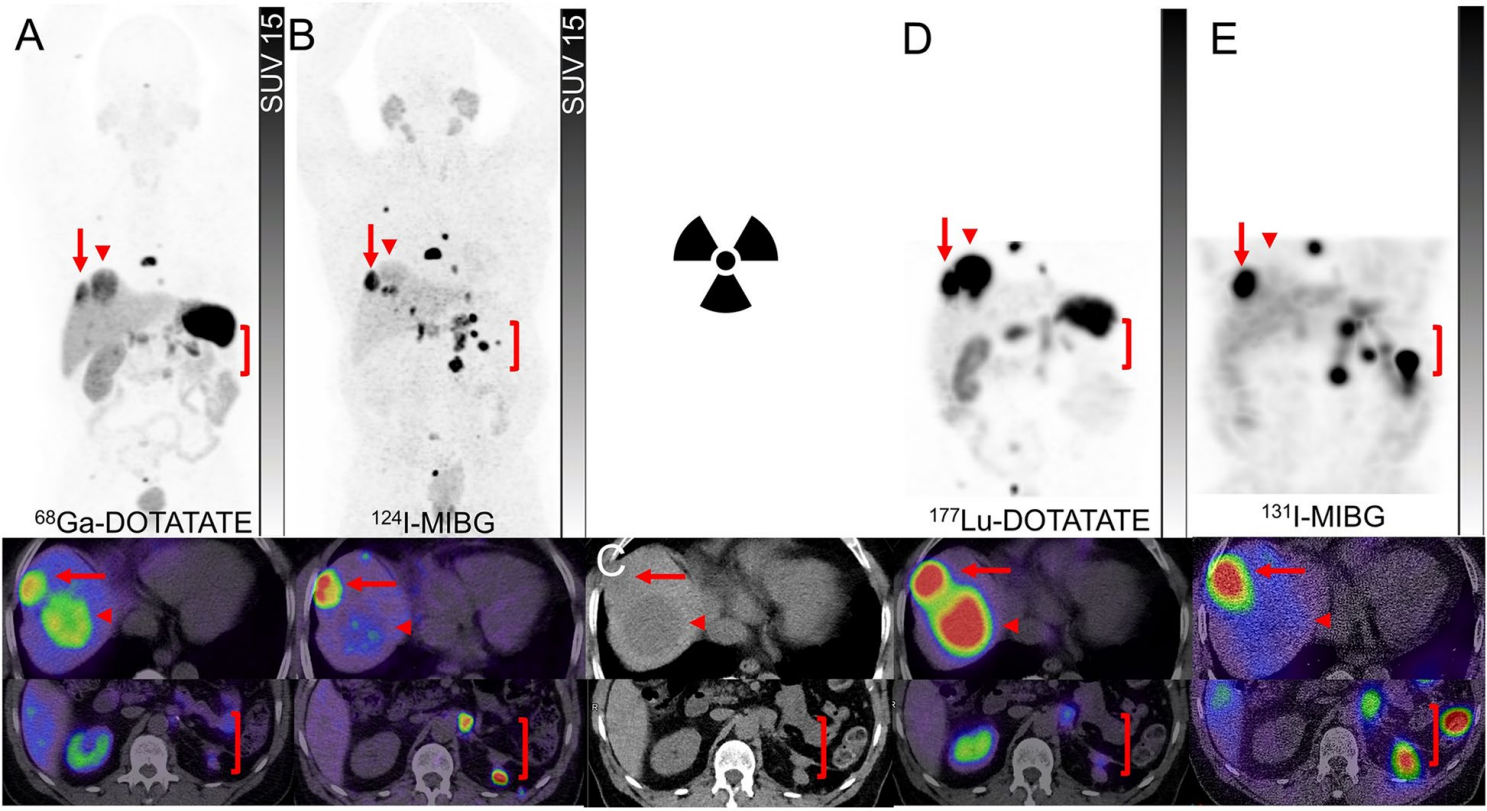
A patient with metastatic pheochromocytoma, previously treated with left adrenalectomy, presented with rapidly rising metanephrines. ^68^Ga-DOTATATE PET and PET/CT(A) showed intense uptake (Krenning score 4) in a thoracic vertebra, two dominant moderately avid (Krenning score 3) hepatic dome lesions (arrows and arrowheads), and mild to moderately avid soft tissue recurrence in the left upper quadrant surgical bed (brackets). ^124^I-MIBG PET and PET/CT (B) showed intense uptake in the bone metastases, variable uptake in hepatic dome lesions [a lesion with mild uptake (arrowheads) and other with intense uptake (arrows)], and intense uptake in the left upper quadrant soft tissue lesions (brackets). Corresponding CT images showed hypodense lesions in the liver (arrow and arrowhead) and soft tissue nodules in the left upper quadrant (bracket) (C). Due to heterogeneity of tracer uptake on both pre-treatment PET scans and the inability to target all sites of disease using a single therapeutic radiopharmaceutical, the patient underwent tandem treatment with 8 GBq of ^177^Lu-DOTATATE and 2.7 GBq of ^131^I-MIBG. Post-treatment ^177^Lu-DOTATATE SPECT and SPECT/CT (D) showed intense uptake in the bone and both hepatic dome metastases (Krenning score 4) (arrows and arrowheads) but only mild uptake in left upper abdomen soft tissue lesions (brackets). Similar to pre-treatment ^124^I-MIBG PET, post-treatment ^131^I-MIBG SPECT and SPEC/CT (E) demonstrated intense uptake in the bone, intense uptake in abdominal left upper quadrant soft tissue lesions (brackets) but in only one of the hepatic lesions (arrows) with no significant uptake in the other (arrowheads), emphasizing the ability to cover disparate molecular imaging phenotypes by using a combination of therapeutic radiopharmaceuticals
